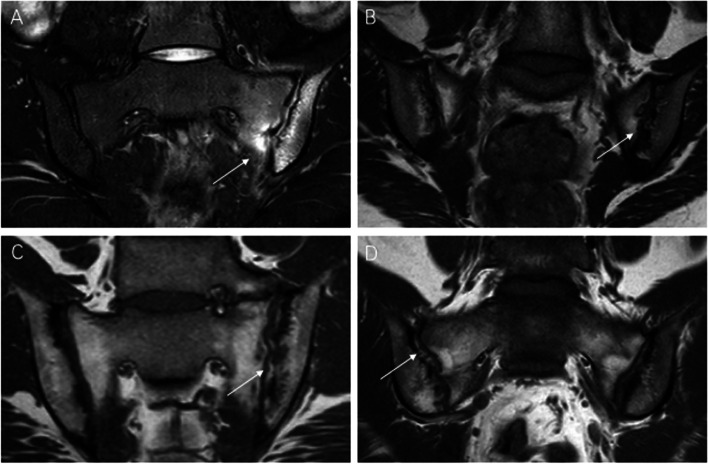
MRI findings of active inflammation and chronic structural damage in axSpA. A Bone marrow edema on the left side of sacroiliac joints. B Erosion of the left iliac bone and sacral bone. C Fat metaplasia in the bilateral sacroiliac joints. D Backfill in the right sacroiliac joints. MRI: magnetic resonance imaging; axSpA: axial spondyloarthritis
